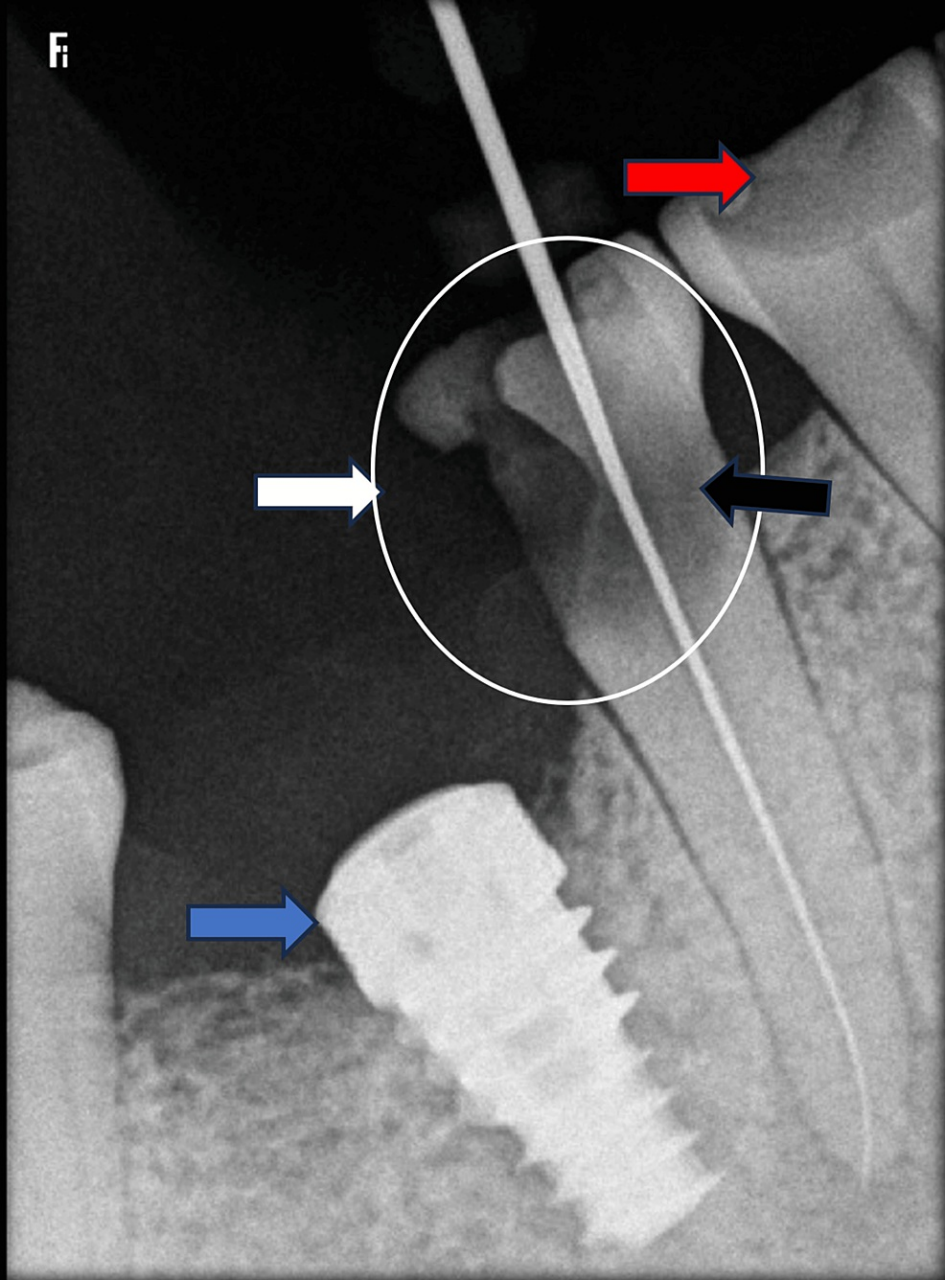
Radiographic interpretations Crownless implant in tooth 46 (blue arrow); Disto-proximal Caries in tooth 45 (white arrow and circle); Erosion in tooth 45 (black arrow); and Erosion in tooth 44 (red arrow).
